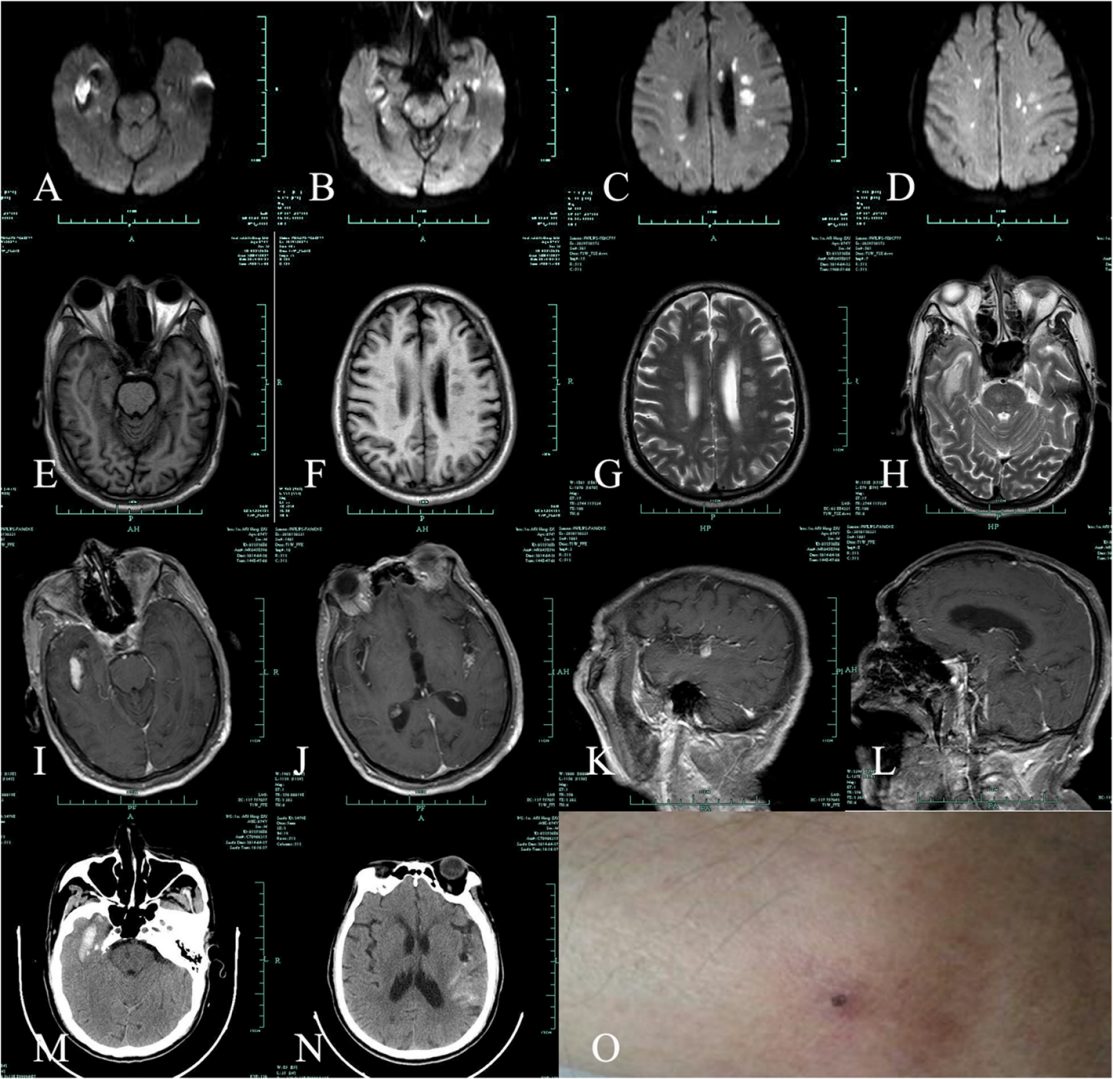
An initial cranial MR image showing hemorrhage in the right temporal lobe, left temporal parietal subarachnoid, and multiple infarcts throughout the deep white matter. a-d: A diffusion-weighted image (DWI) shows increased intensity in central of the lesion with reduced intensity surrounding the lesion in the right temporal lobe. Multiple hyperintense lesions can be seen in the brain stem, bilateral cerebral, and cerebellar hemispheres. e-f: T1-weighted image (T1WI) shows isointensity lesions in the right temporal lobe and multiple hypointense lesions throughout deep white matter. g-h: T2-weighted image (T2WI) shows hyperintense areas in the right temporal and multiple hyperintense lesions throughout the deep white matter. i-l: enhanced T1-weighted image shows no enhanced lesions or dural meningeal enhancement. m-n: cranial CT shows right temporal lobe hemorrhage and left temporal parietal subarachnoid hemorrhage. o: an eschar present on the left calf
